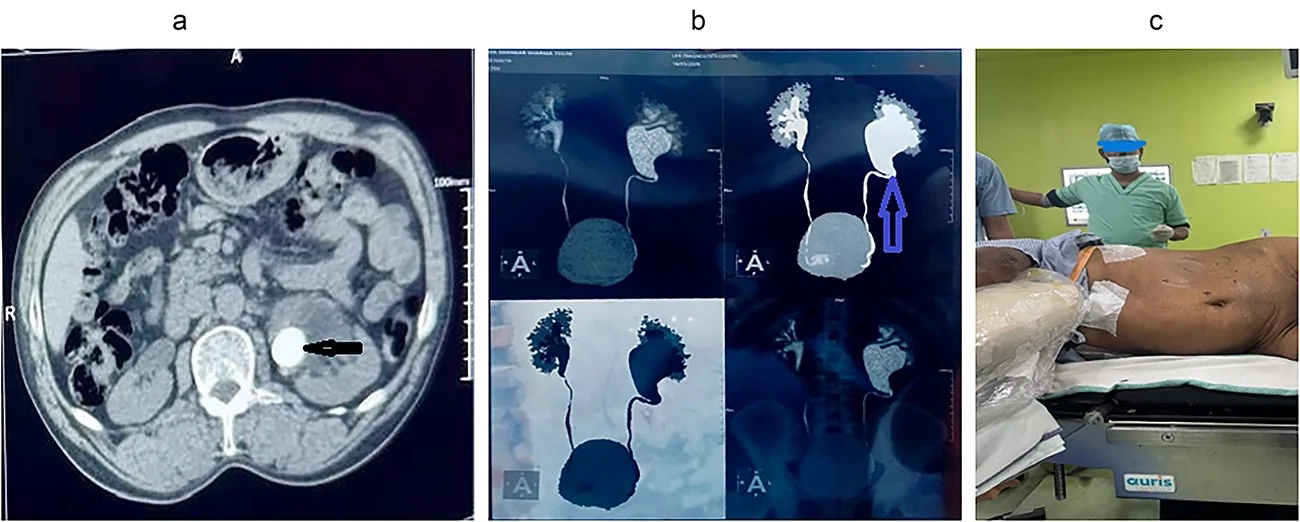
(a) Contrast-enhanced CT urography (coronal section) demonstrating a large radio-opaque calculus measuring 5.2 cm within the left renal pelvis, associated with moderate hydronephrosis (black arrow). (b) CT urography showing preserved bilateral renal excretory function, with contrast outlining the giant calculus occupying the extrarenal pelvis of the left kidney (blue arrow). (c) Patient positioned in a modified left lateral decubitus position, optimized for the transperitoneal laparoscopic approach.
